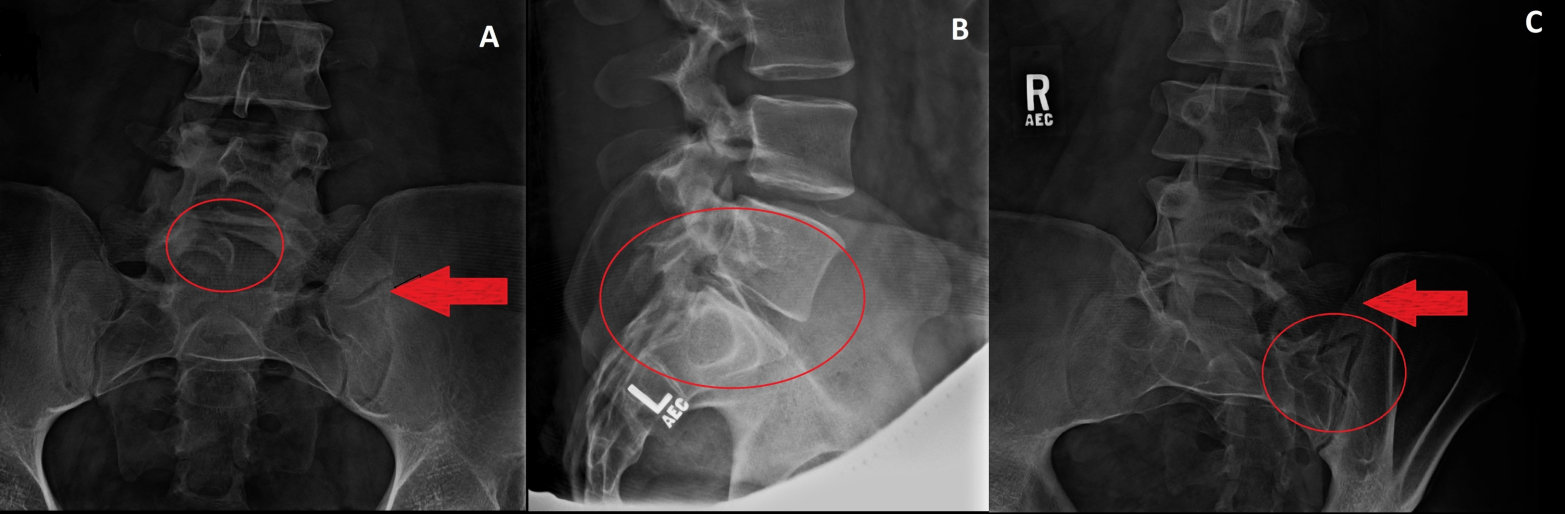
X-ray of LS [A] Anteroposterior (AP) view: The red arrow shows enlargenment of the left transverse process of L5 vertebra forming pseudo-articulation with the sacrum. The red circle shows spina bifida occulta. [B, C] Left lateral and right lateral views: the red arrow [C] shows enlargement of the transverse process of L5. The red circles [B, C] demonstrate pseudo-articulation of the L5 transverse process with the sacrum.
